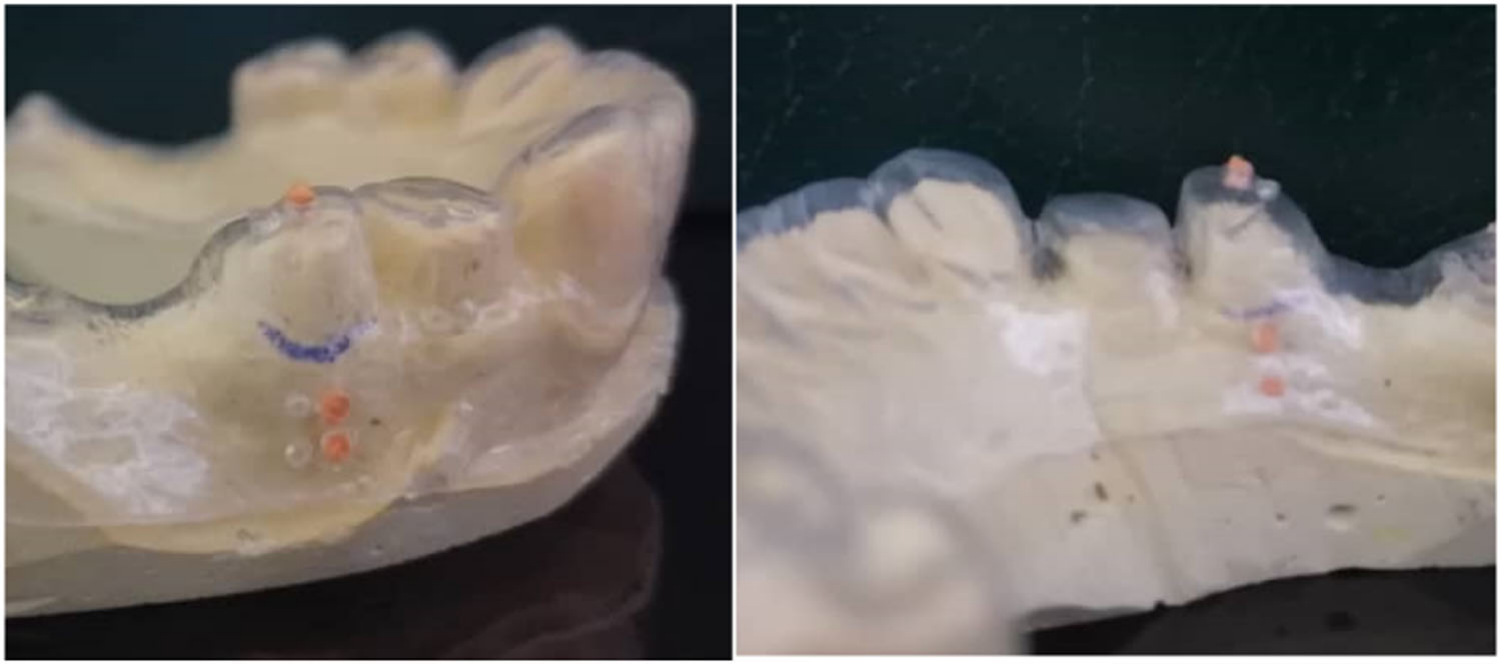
The stent's standardized holes for clinical and radiographic measurements.
